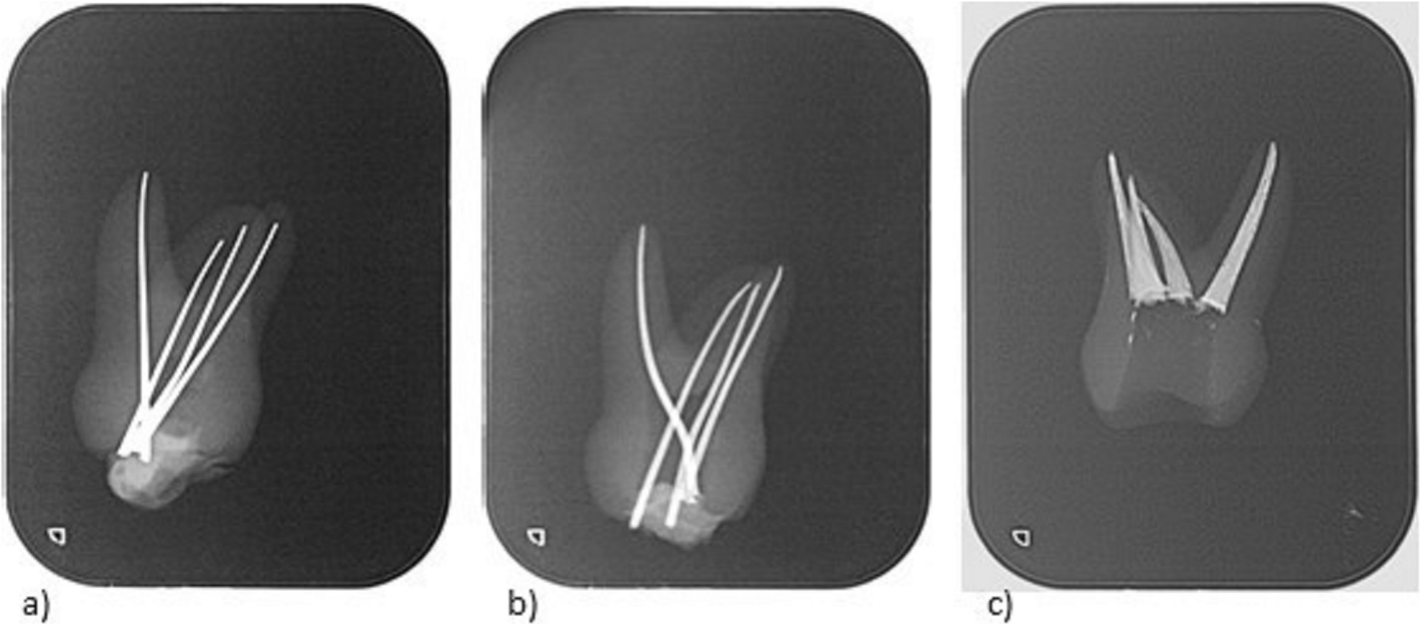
Radiographs of a maxillary first molar DRSK RCT tooth model: a lengths measuring with silverpoints, b control of gutta-percha master cones, c control of the completed root canal filling
